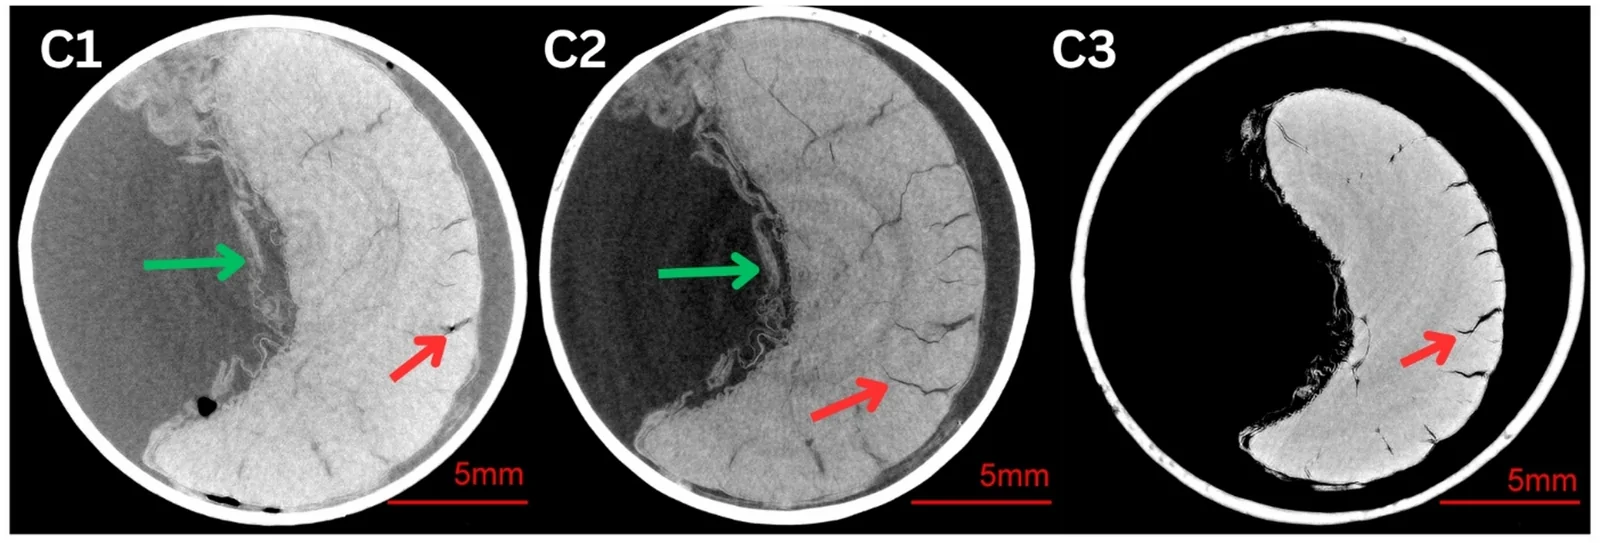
Axial CT slices of a sample container containing C1: 70% ethanol fixed SDFT, submerged in 70% ethanol, C2: 70% ethanol fixed, 100% ethanol dehydrated SDFT submerged in 100% ethanol and C3: air dried SDFT after previous processing. Scale bars = 5 mm. Green and red arrows depict loose connective tissue and cracks respectively.
